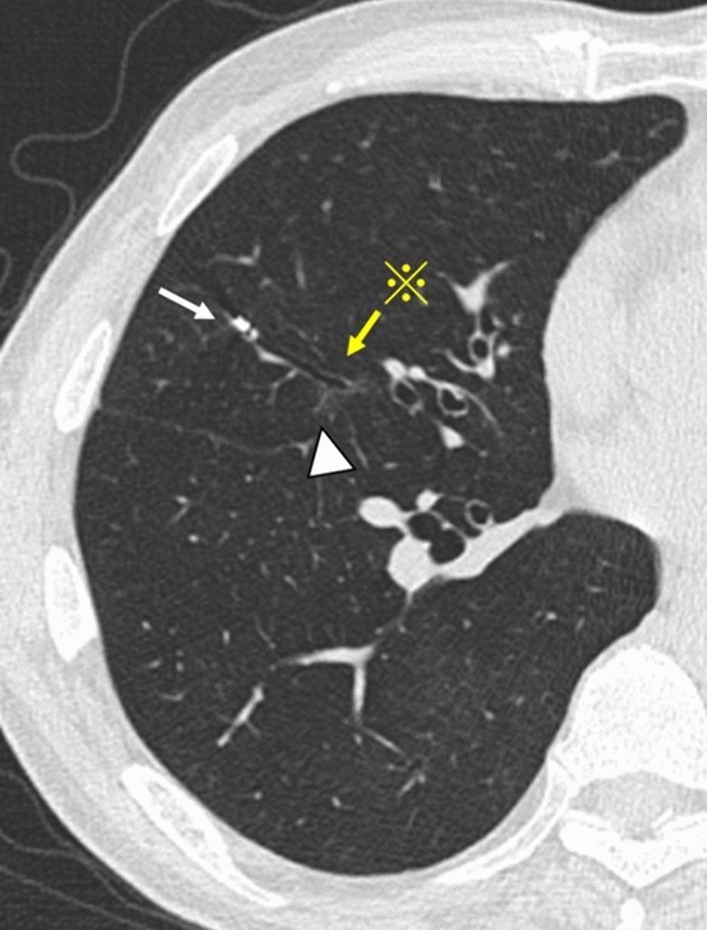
Deviation between the targe placement site and the actual placement site. The upper margin and ventral side of the tumour (arrowhead) were set as the target placement sites (asterisk, yellow arrow). The actual RFID tag placement site is distal to the target site (white arrow). The distance from the RFID tag to the target site was 20.8 mm, which was judged to be ‘incomplete.’ *RFID* radiofrequency identification, *CT* computed tomography, *GGN* ground-glass nodule, *RFID* radiofrequency identification, *VBN* virtual bronchoscopic navigation.
